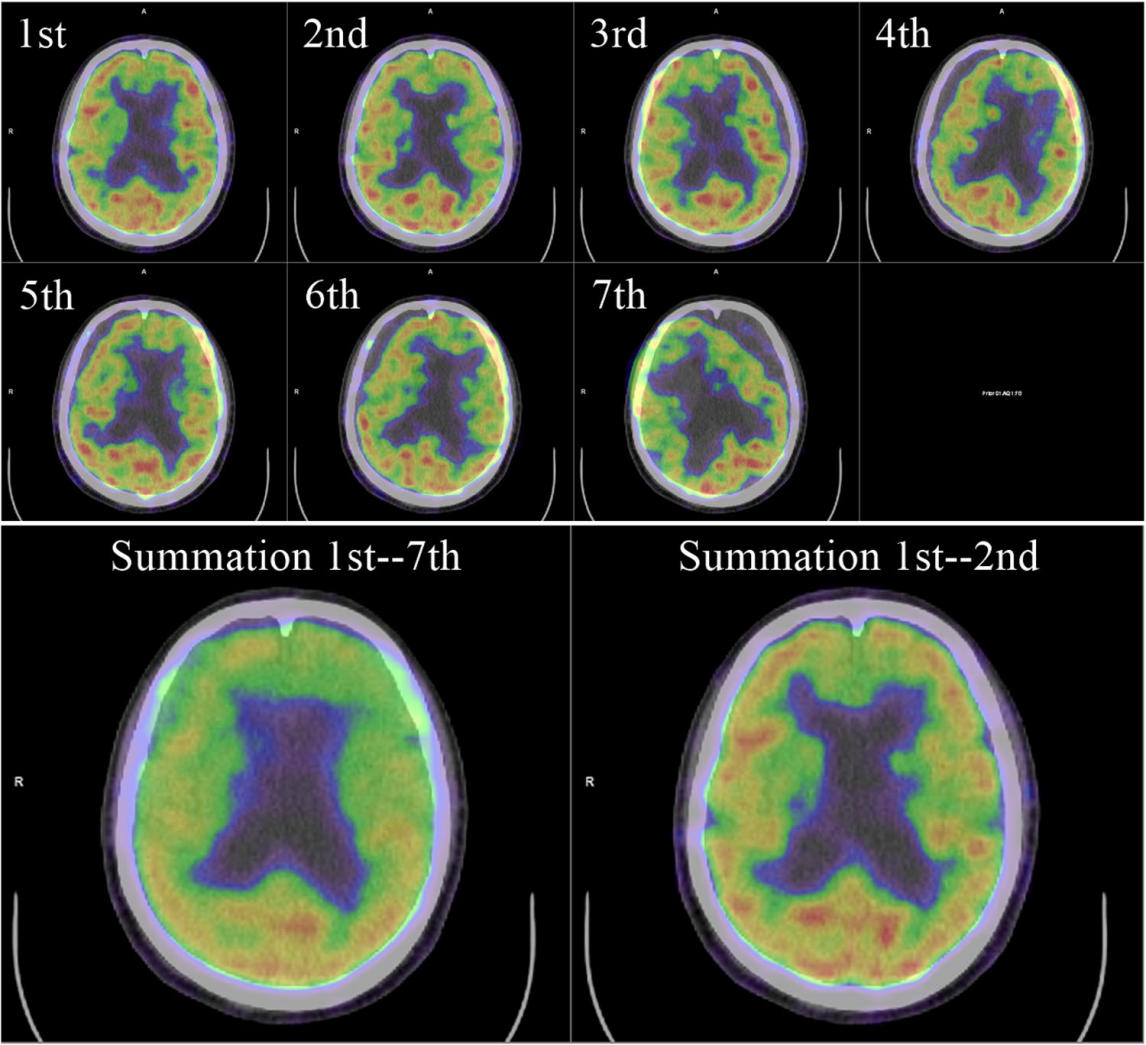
Axial images of serial whole-body dynamic^18^F-FDG PET/CT in a case undergoing cancer screening. The patient slightly rotated his head on    the 3rd  scan and largely rotated it on the 4th and 7th   scan (upper and middle row). Although the contour of cerebral construction is unclear and apparent laterality and anteroposteriority of cerebral glucose metabolism due to error of attenuation correction is shown in the image of 1st–7th summation (lower left), the summation of the 1st–2nd images shows the cerebrum clearly and error of attenuation correction is corrected (lower right)
